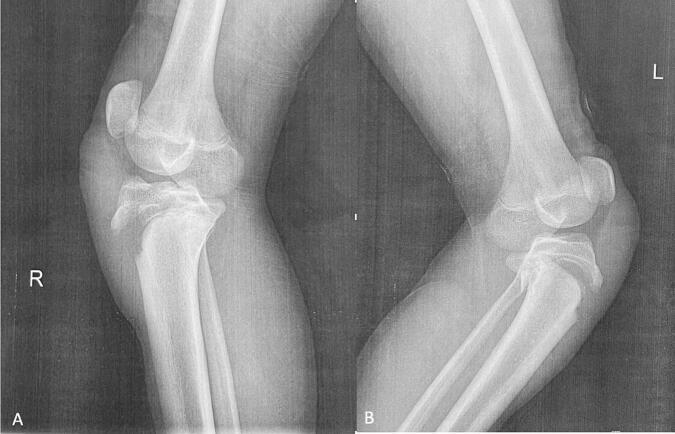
Pre-operative lateral plain radiographs of both knees. (A) Right tibial tubercule fracture, Ogden type lll. (B) Left tibial tubercule fracture, Ogden type lV.
